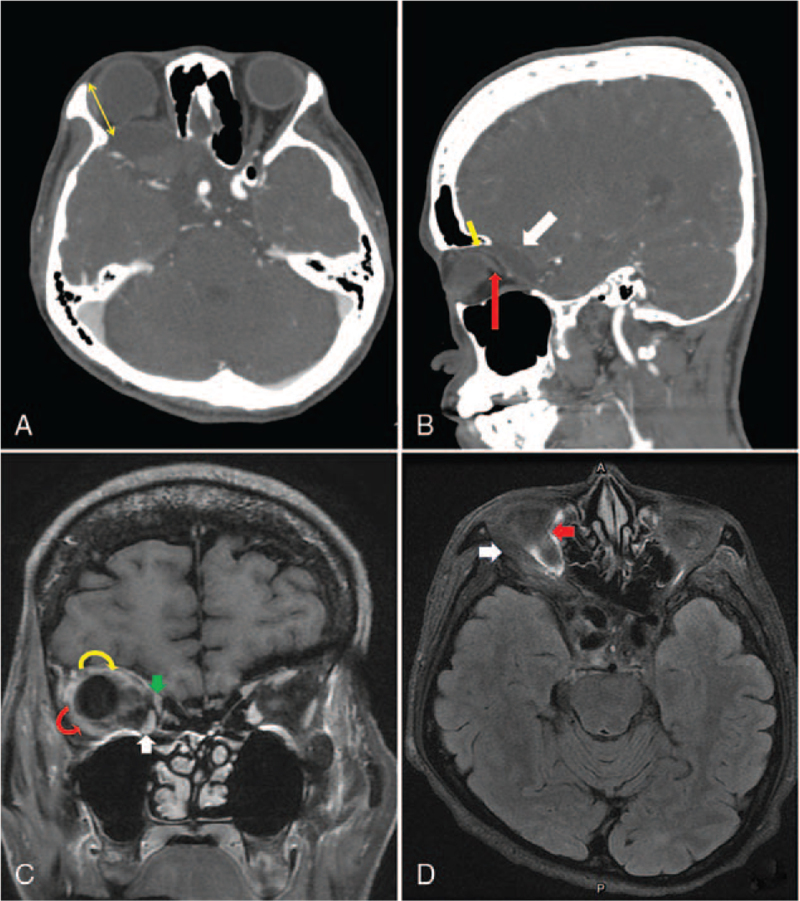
(A) A short lateral orbital wall (yellow double head arrow) and sphenoid wing dysplasia of the right orbit are noted. (B) A meningocele protruding through the orbital defect into the right orbit and lifting the globe (white arrow). The herniated meningocele compresses the posterior portion of the superior rectus muscle (SR) (yellow arrow) and optic nerve (red arrow). (C) Brain magnetic resonance imaging (MRI) showing inferior displacement of the lateral rectus muscle (LR) (red curved arrow) and medial displacement of the SR (yellow curved arrow) with a supra-temporally shifted globe. The medial rectus muscle (green arrow) and inferior rectus muscle (white arrow) are hypertrophic and crowded in the medial orbital cavity. (D) The meningocele herniates into the right orbit and compresses the LR (white arrow). The LR is shifted inferiorly and appears at the same level as the inferior rectus muscle (red arrow).
